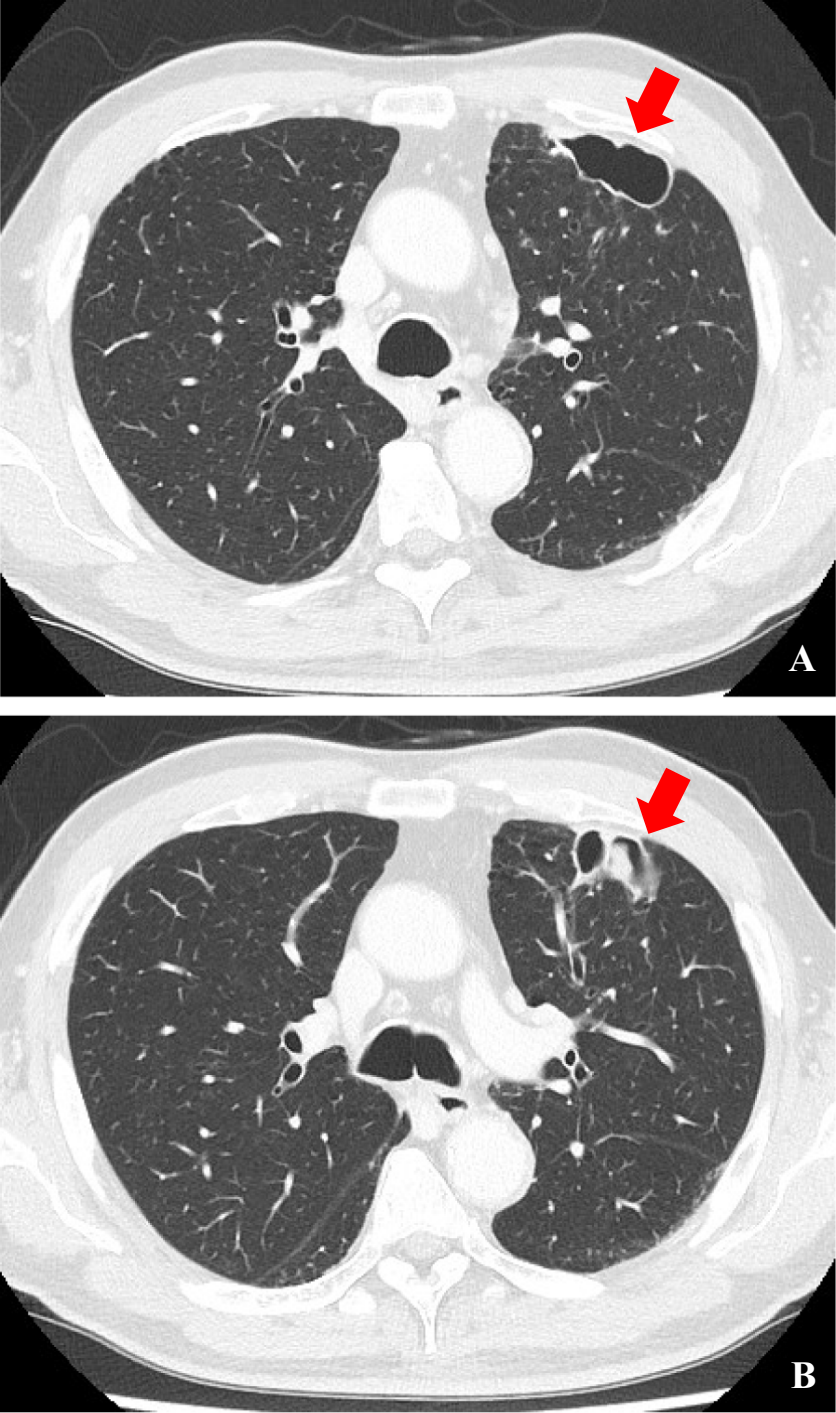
Computed tomography scan of the thorax. The left upper lobe of the lung has a cavity (A, B) and internal nodule (B); these are indicated using red arrows
